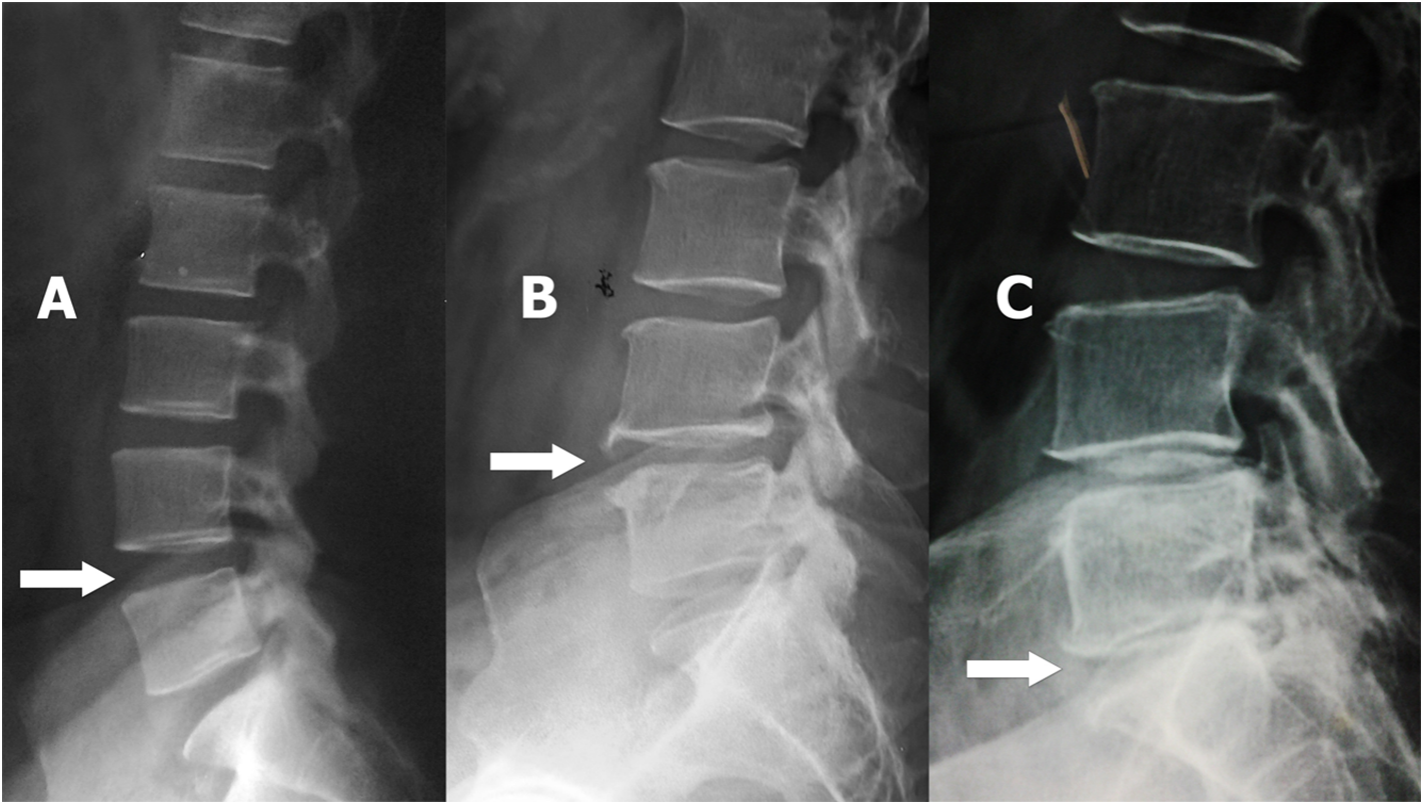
Assessment of the x-ray features of lumbar disc degeneration—lateral x-ray of lumbar spine. Arrows—A–no disc space narrowing/anterior osteophyte (grade 0 lumbar disc degeneration), B–mild disc space narrowing and small anterior osteophyte (grade 1 lumbar disc degeneration), C–small anterior osteophyte and moderate disc space narrowing (grade 2 lumbar disc degeneration) [3].
